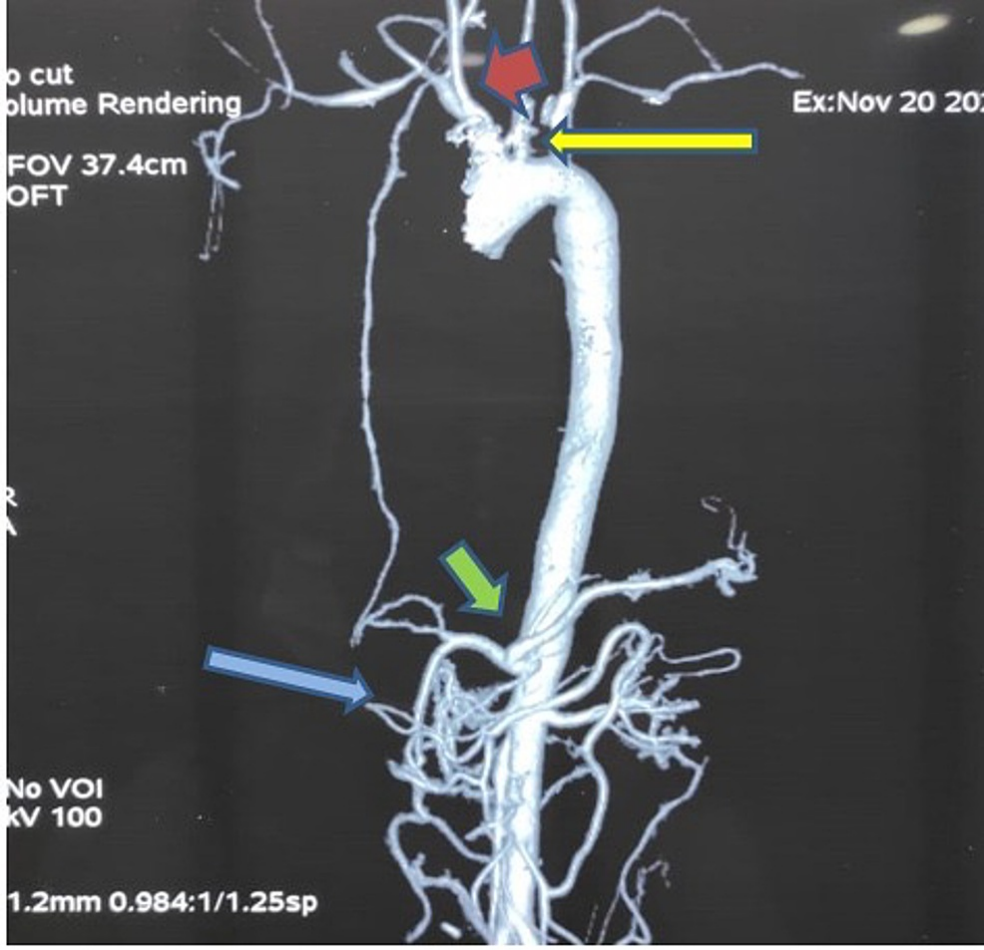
CT angiogram coronal view. The red arrow shows narrowing of the right internal carotid artery (a), the yellow arrow indicates narrowing of the origin of the right subclavian artery (b), the green arrow indicates narrowing of the origin of celiac trunk (c), and the blue arrow indicates the narrowed origin of the superior mesenteric artery, and its beaded appearance (d)
